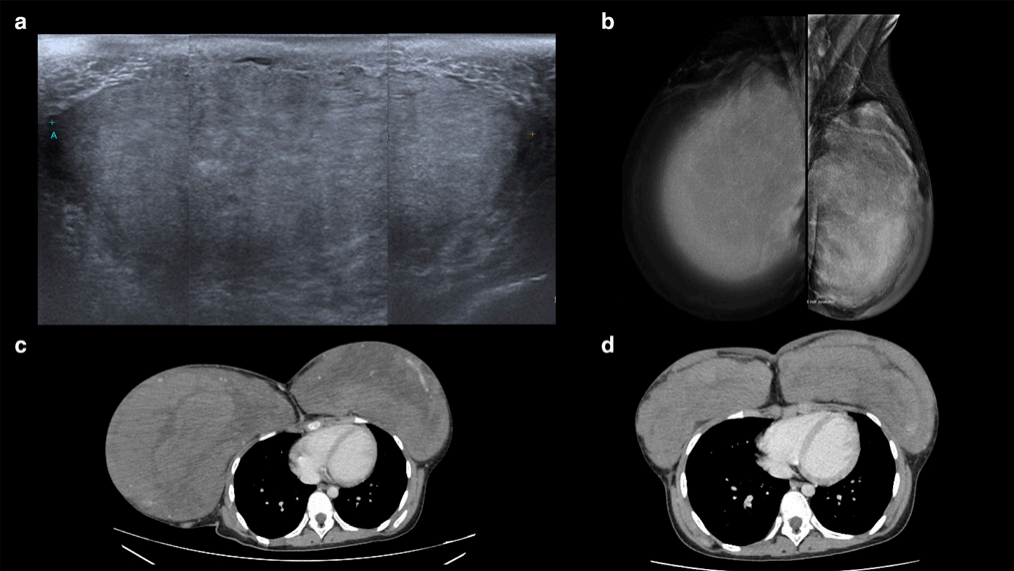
(A-D) An 11-year-old girl with bilateral breast enlargement. (A) Breast ultrasound shows a large well-defined heterogeneous mass in the right breast, measuring 15 cm. (B) On mammogram, MLO view, the breasts are extremely dense and diffusely enlarged. (C) Axial preoperative chest CT shows a large heterogeneously enhanced mass occupying each entire breast, more prominent on the right. (D) These masses re-enlarge 4 months following reduction mammoplasty.
